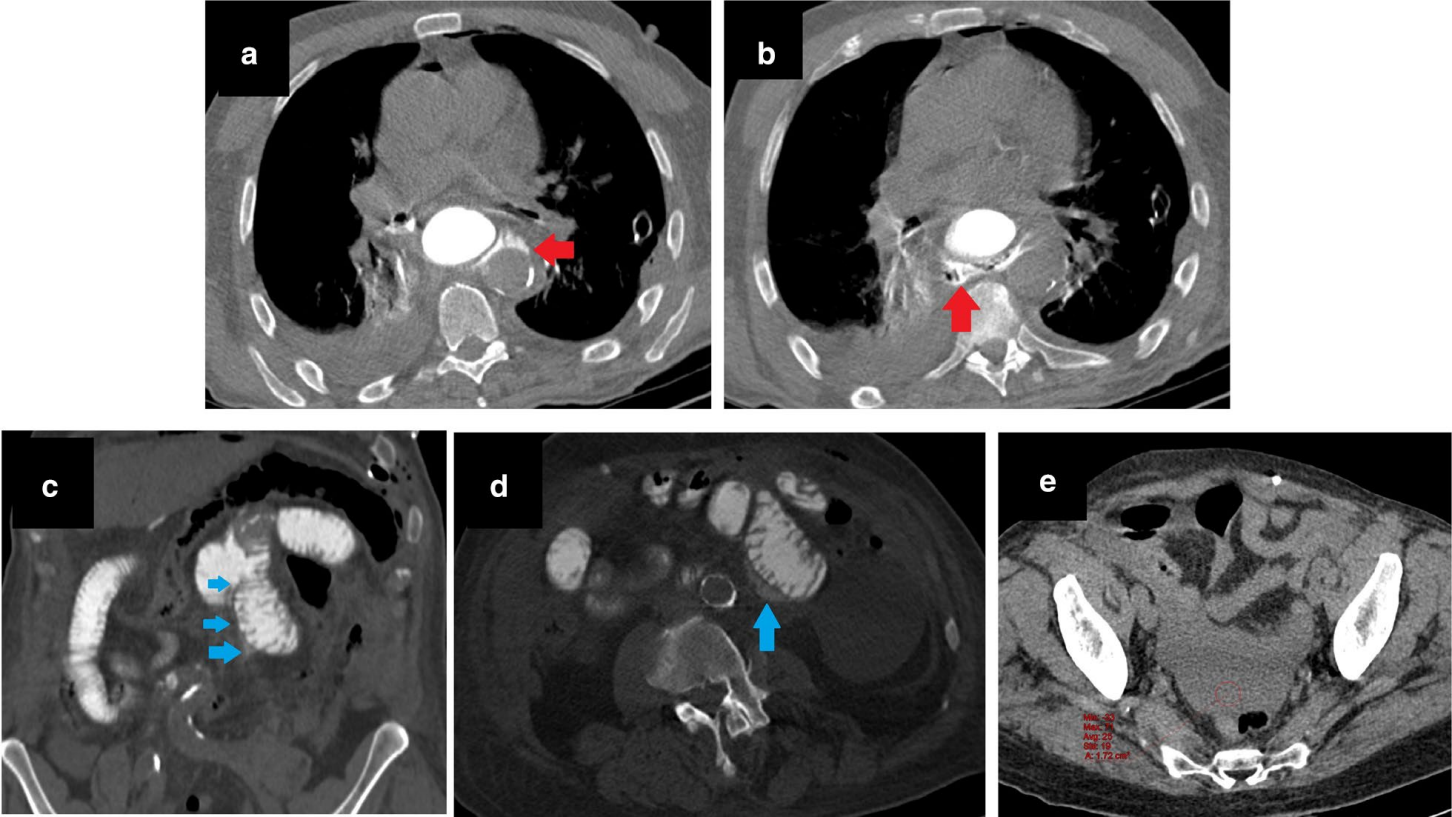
Satisfaction of search (type 10) error. A patient with gastric cardia tumor underwent total gastrectomy and distal esophagectomy. On the first postoperative day, dyspnea and abdominal pain developed. Anastomosis leakage was suspected, and thoracoabdominal CT with oral contrast agent was performed. The on-call radiologist noticed apparent contrast extravasation from esophagojejunostomy to the posterior mediastinum. The study was reported as “consistent with anastomosis leakage from esophagojejunostomy” (red arrows, a, b). Due to the “satisfaction of search” effect of this significant finding, the remainder of the examination was reported as normal. However, the patient’s abdominal complaints progressed, and the general condition of the patient deteriorated, so the patient was re-operated on, and additional jejunojejunostomy leakage was detected. When the preoperative CT was retrospectively evaluated, apparent signs of leakage in the jejunojejunostomy area were noticed. Linear hyperdensity consistent with leakage extending inferiorly from the anastomosis (blue arrows, c), extraluminal contrast material between jejunal loops (blue arrow, d), and pelvic fluid with relatively high density (red ROI mark, e) were overlooked findings
